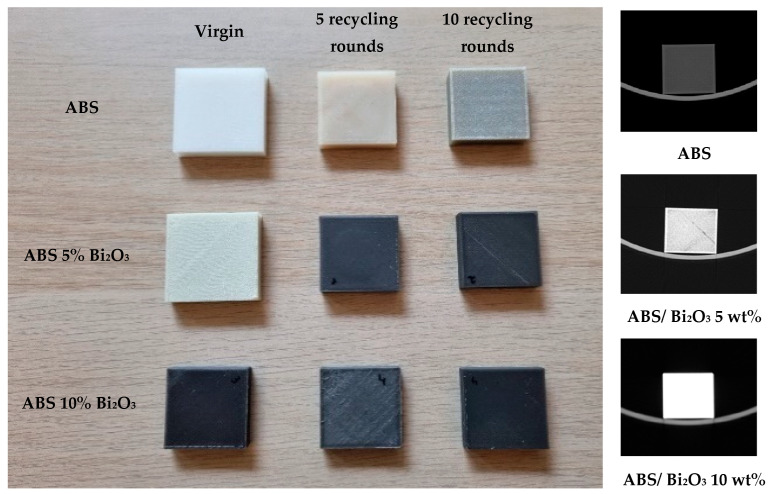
Visual appearance of 3D-printed samples after various recycling rounds (Left) and CT scans of virgin samples (Right).
